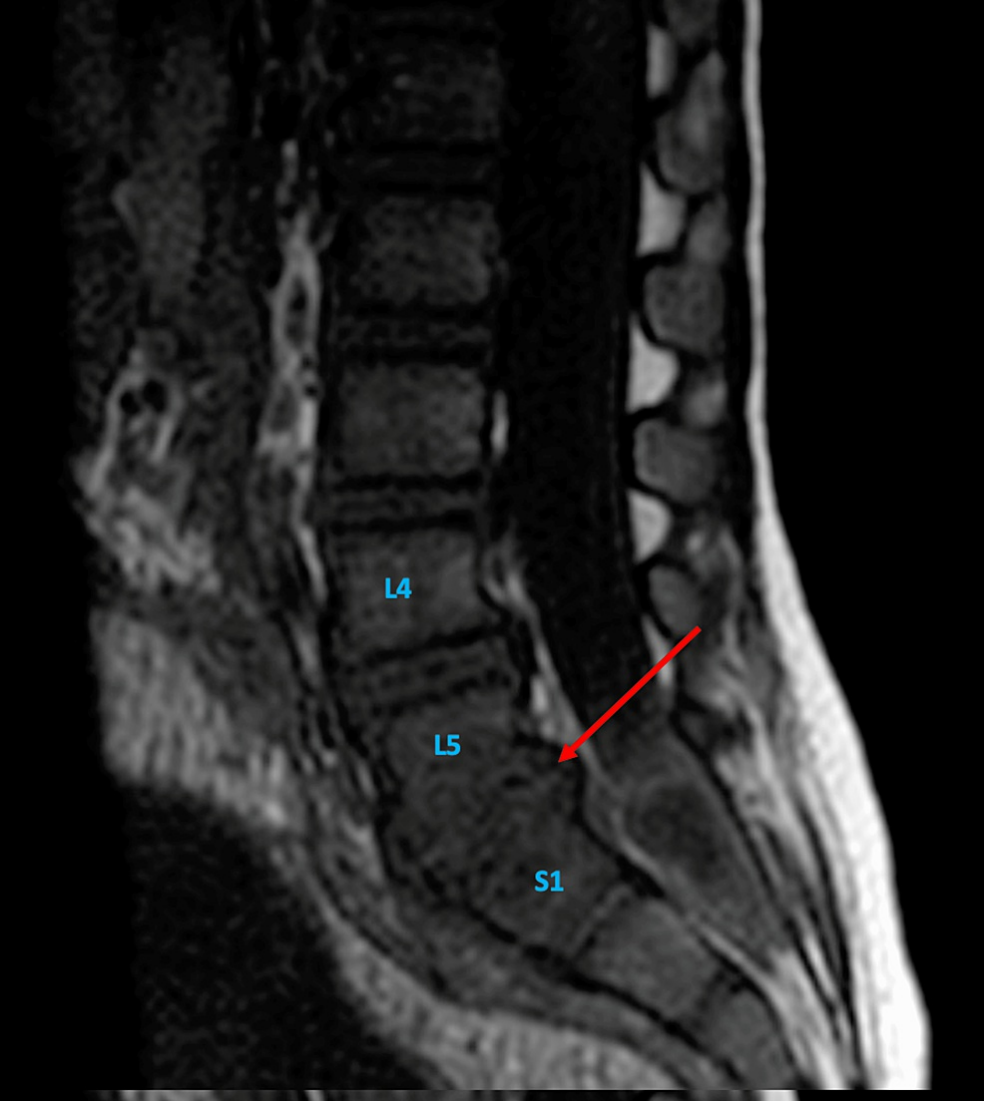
Sagittal T1-weighted MRI of the lumbar spine showing a hypointense marrow signal at L5 and S1 vertebral levels (red arrow).
